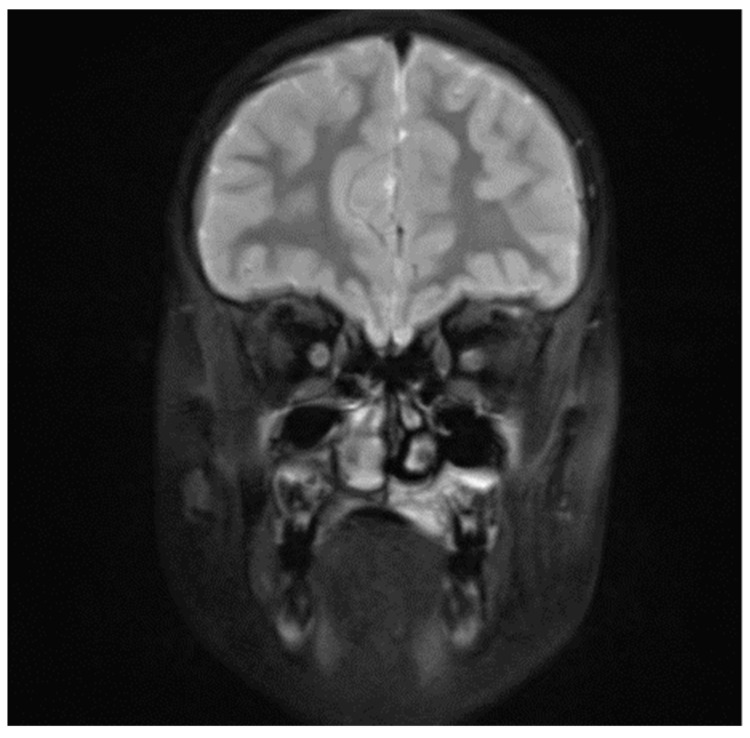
Patient, 8 y.o.: Coronal section MRI T2 fast spin fat-saturation image, increase in signal intensity with thickening of the palatal mucosa on the left side.
